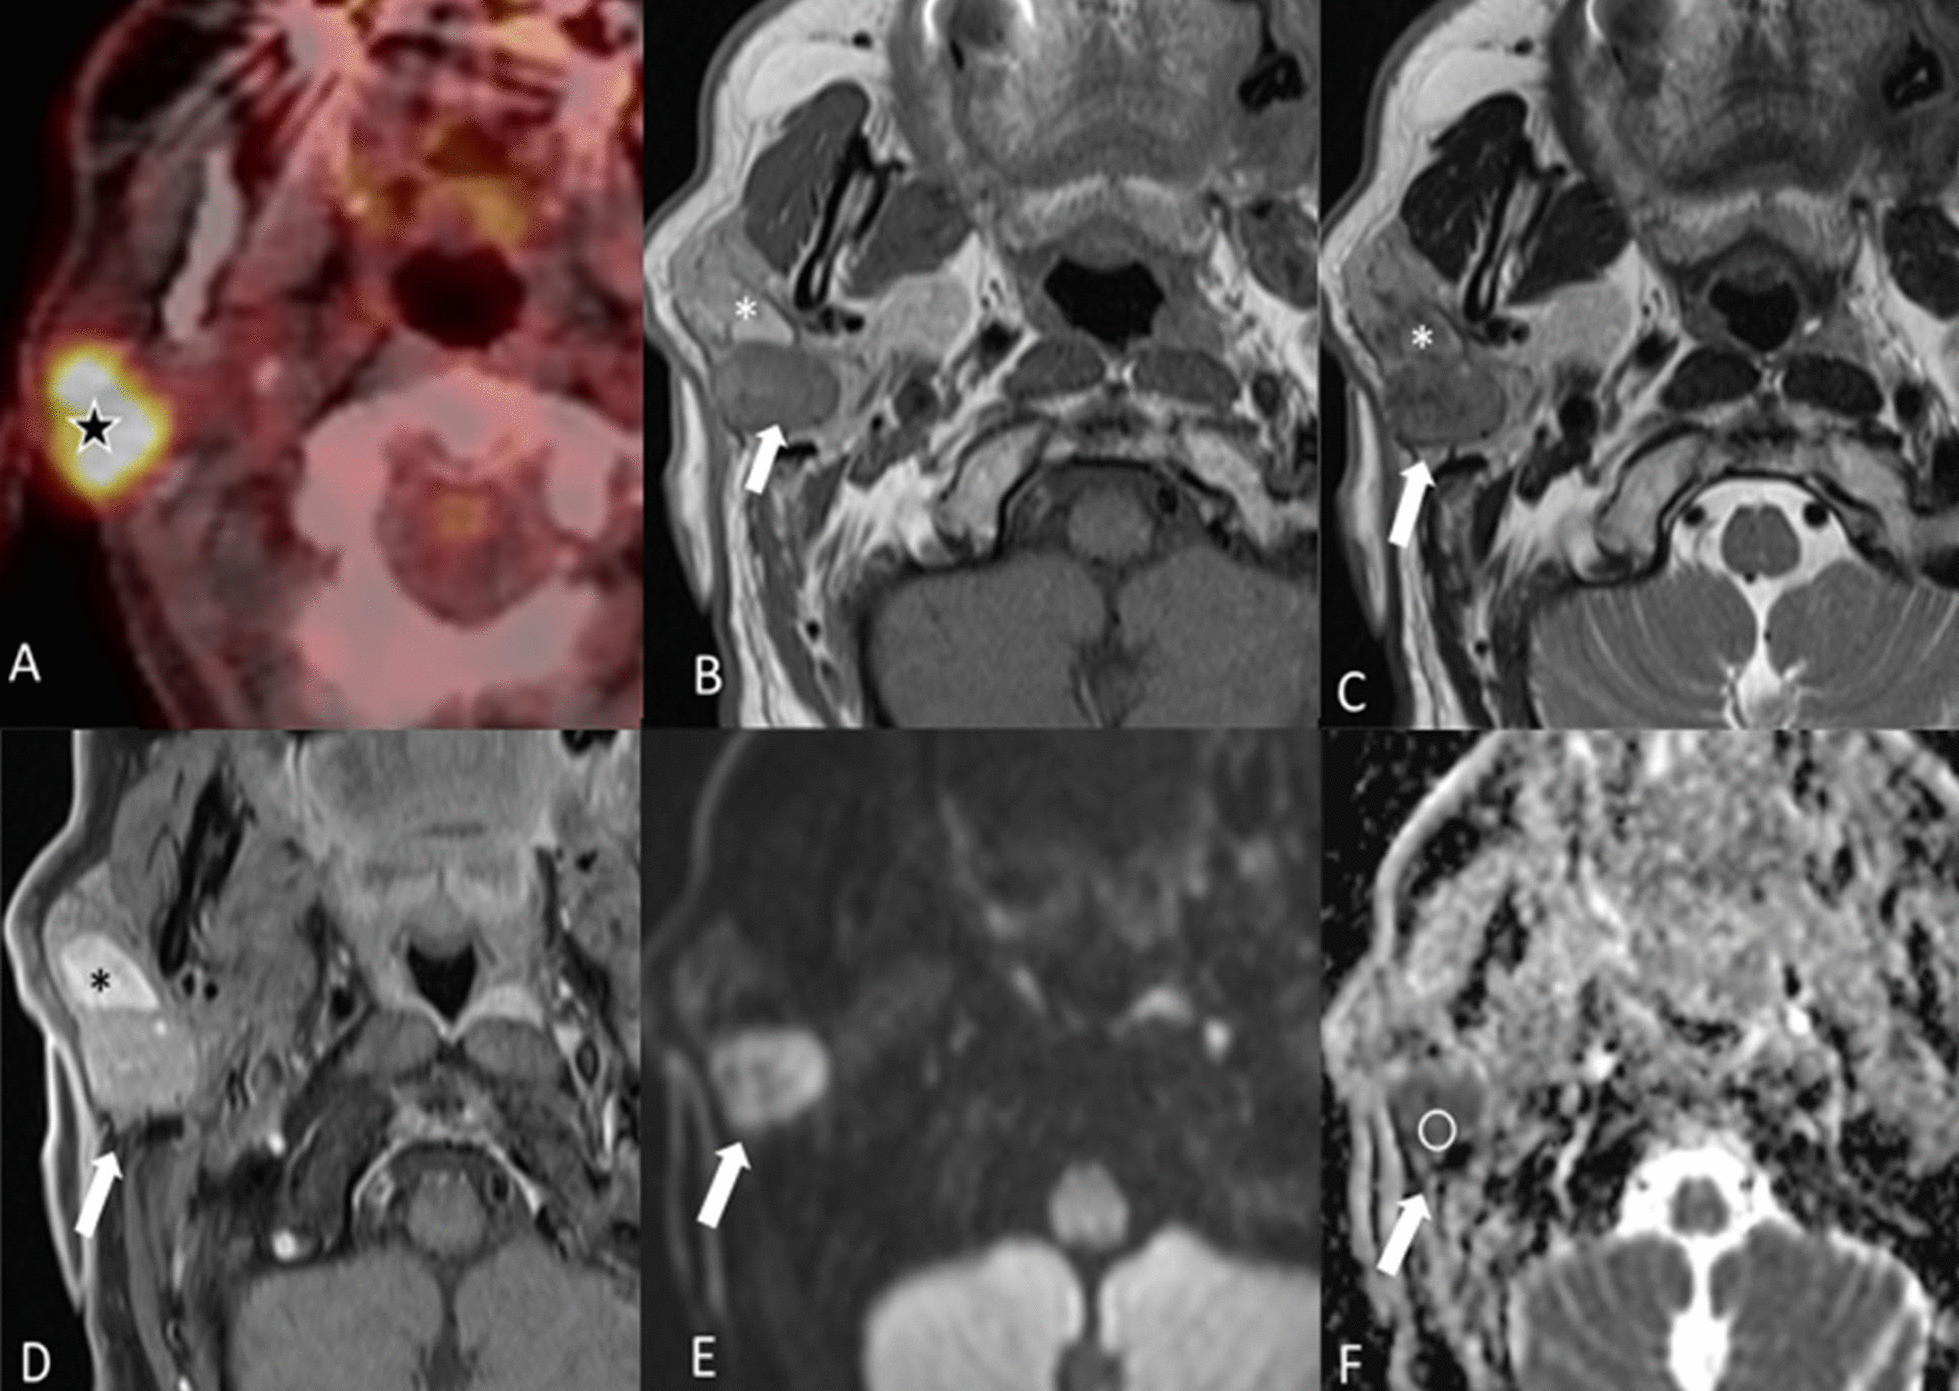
Warthin’s tumor. A 73-year-old male with malignant melanoma of the back who underwent an ^18^F-FDG PET/CT scan for staging. The PET/CT scan (A) showed a highly metabolic lesion in the right parotid gland (star). Fine needle aspiration cytology revealed a Warthin’s tumor. The dorsal part of the lesion is predominantly hypointense on both T1-w axial MRI (B) and T2-w axial MRI (C) with slightly heterogeneous enhancement after injection of contrast as seen on the post-contrast fat saturated T1-w image (D). There is diffusion restriction of the enhancing part with a hyperintense signal on the high b value diffusion-weighted image (E) and a low signal on the ADC map (F). The ventral portion of the lesion (white asterisk) is hypointense on T2-w and hyperintense on T1-w due to some bleeding caused by the fine needle aspiration cytology, which had been performed prior to the MRI
